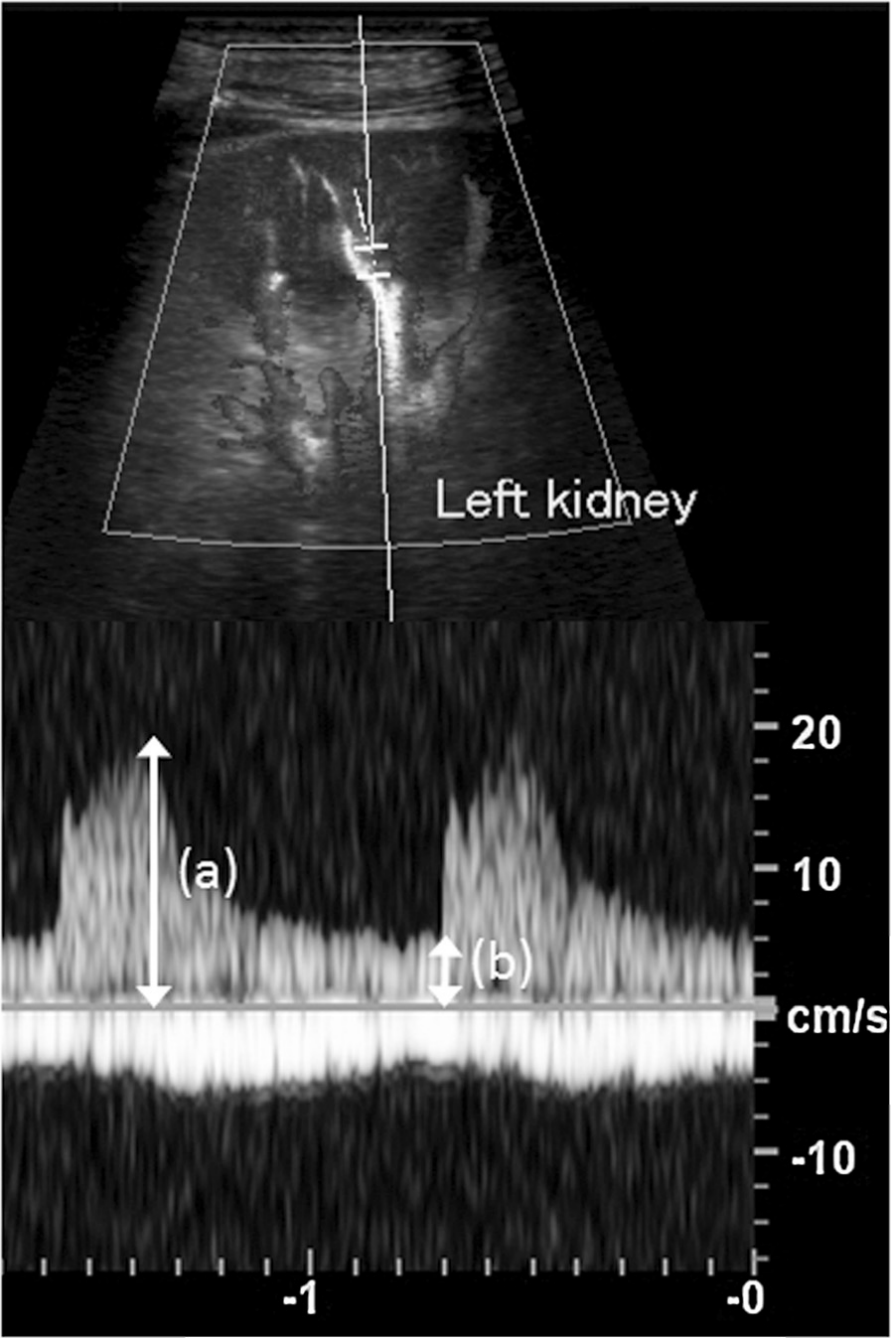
Example of renal resistive index measurement. Pulsed Doppler ultrasonography was obtained in the intra-renal artery at the level of the corticomedullary junction. Renal resistive index is measured as {peak systolic velocity (a) - end diastolic velocity (b)} / peak systolic velocity (a).
